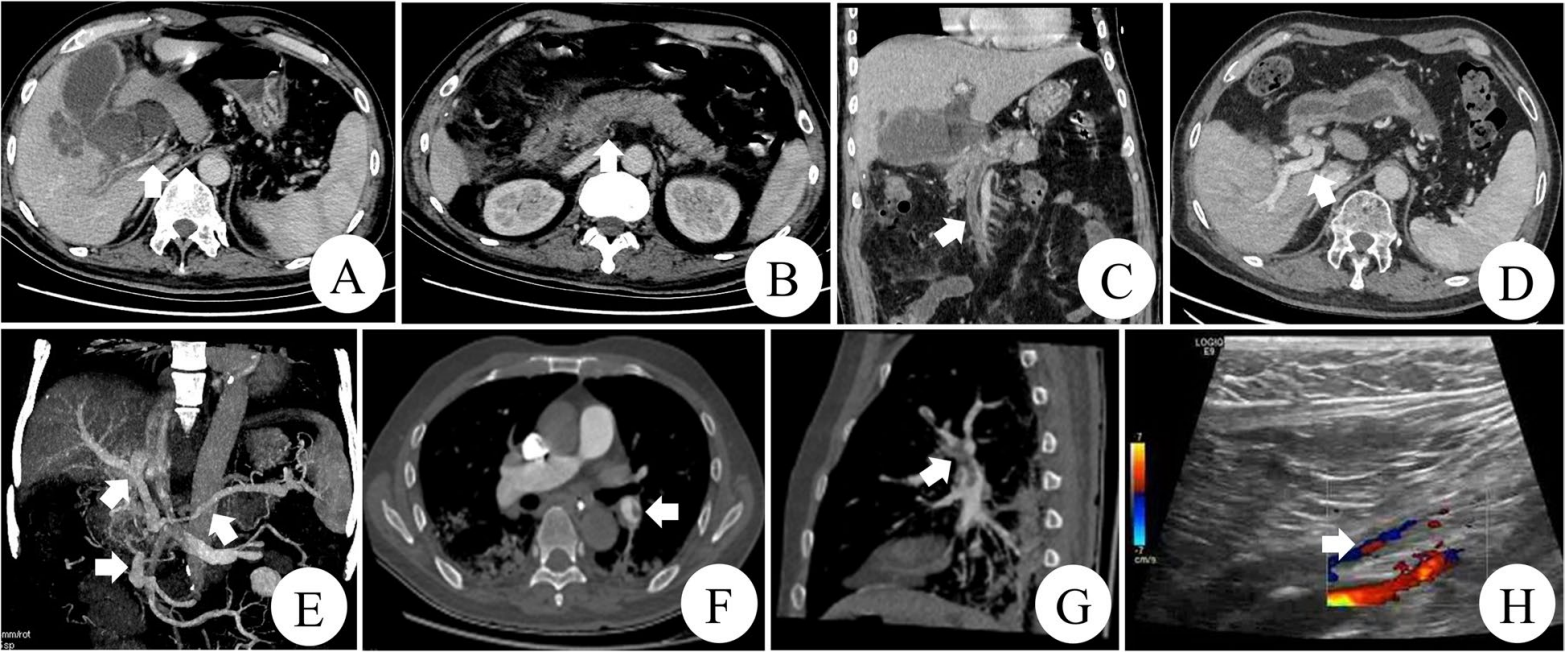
A triphase abdomen computed tomographic revealed filling defect in the major portal vein (A) and a low-density area in splenic vein (B). Coronal reconstruction of the abdominal computed tomography exhibited a low-density area in superior mesenteric vein (C). After 2 years of anticoagulation treatment with oral dabigatran, a triphase abdomen computed tomographic demonstrated complete recanalization of the portal vein thrombosis (D) and coronal maximum intensity projection showed the formation of aberrant collateral vessels and partial recanalization of the splenic vein, superior mesenteric vein thrombosis (E). At admission, transverse (F) and sagittal (G) computed tomography pulmonary angiography demonstrated filling defect in the left pulmonary artery. Color Doppler ultrasound showed thrombosis in bilateral femoral vein and the left popliteal vein
